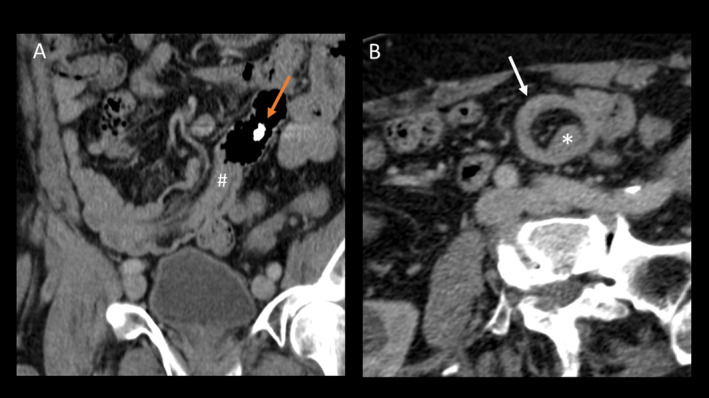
Contrast enhanced abdominal computed tomography (90 mL ACCUPAQUE^TM^ 300 mg) shows the intestinal intussusception (#) in the coronal plane together with the stuck video capsule (orange arrow in A). Axial images resemble the typical target sign appearance (white arrow) of the invaginated ileum (* in B).
